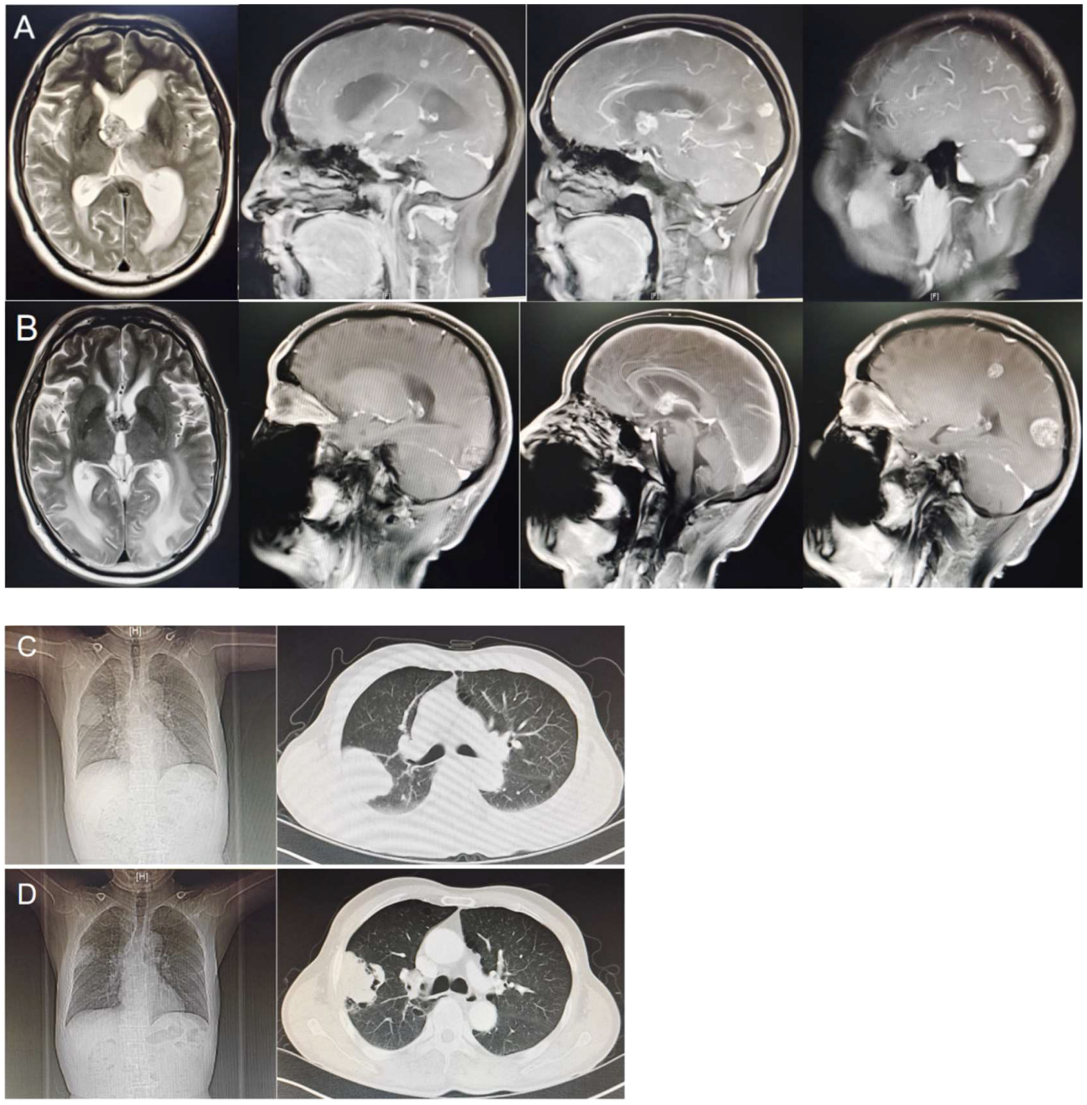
(A) Magnetic resonance imaging showed ventricular occupancy with hydrocephalus (15 January 2022). (B) Magnetic resonance showed leukoedema even worse, brain metastasis overall progress, and meningeal signal enhancement than before (15 September 2022). (C) A computed tomography scan showed a soft tissue mass in the upper lobe of the right lung (18 January 2022). (D) Computed tomography scan showed the primary lesion of the right lung was smaller than before and accompanied by cavity formation (05 September 2022).
